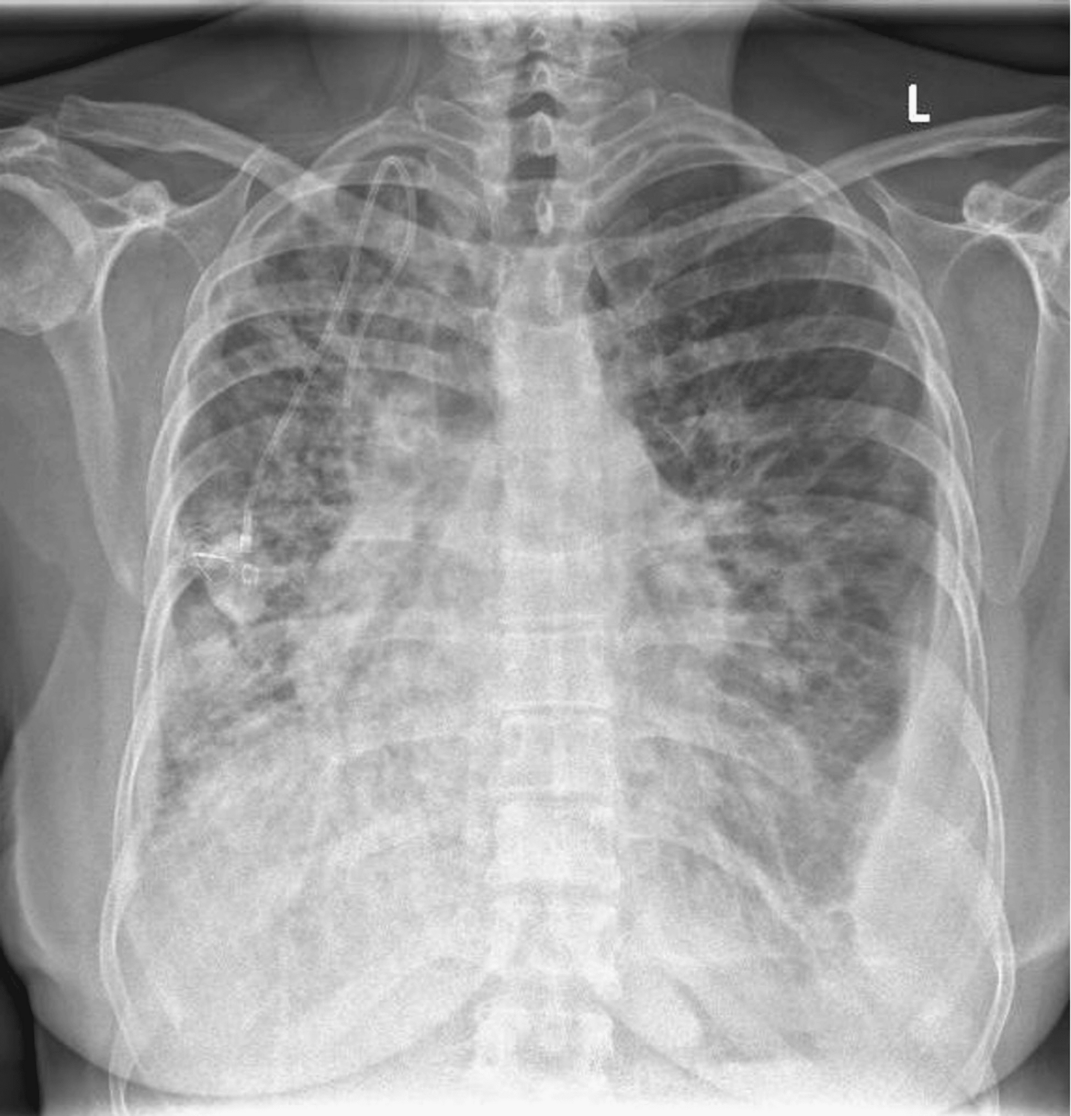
Chest radiograph showing interval development of bilateral hazy infiltrates over both lung fields
